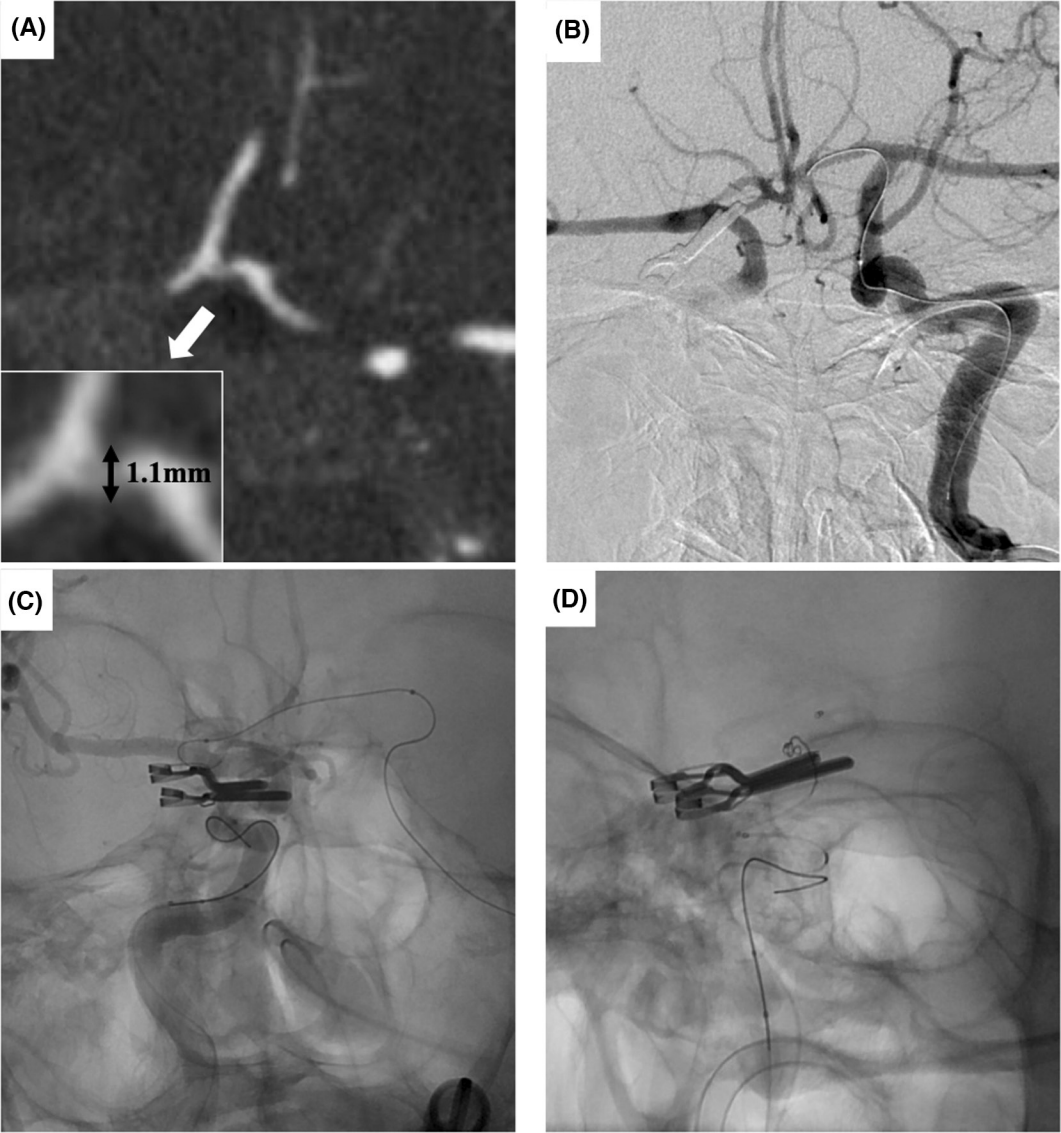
Axial computed tomography angiography source images (A) show anterior communicating artery with a diameter of 1.1 mm. Left internal carotid artery (ICA) angiography (B) under balloon inflation in the right proximal ICA and the fluoroscopic view (C and D) show retrograde navigation of the stent delivery catheter to the contralateral horizontal segment of the anterior cerebral artery (B), ipsilateral terminal ICA (C), and right fetal variant posterior cerebral artery (D) from the contralateral ICA.
